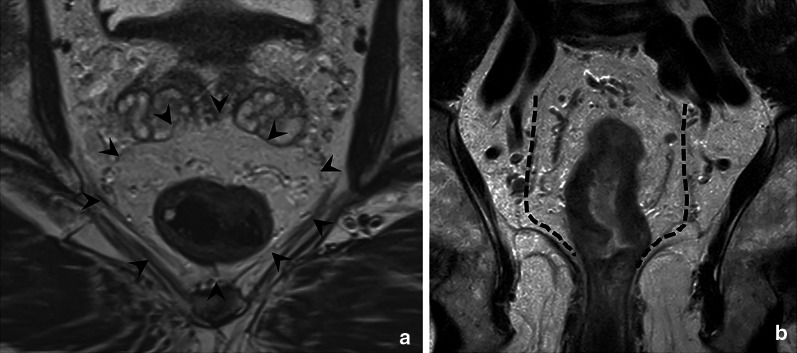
T2-weighted axial MR image a of a male individual demonstrating the mesorectal fascia as a thin hypointense line (arrowheads) surrounding the mesorectal compartment. Coronal image b demonstrating the distal tapering of the mesorectum
